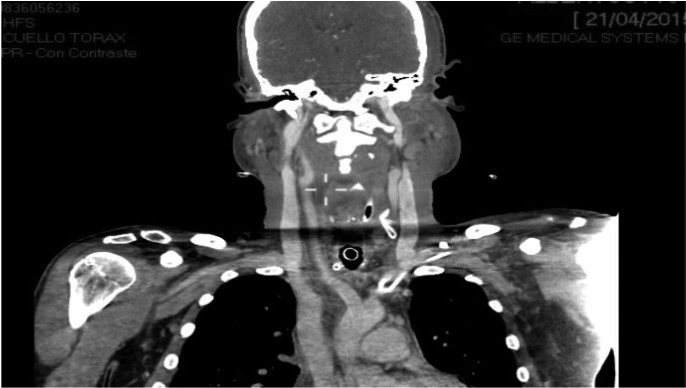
CT images that show the pus which spread from the tooth 48 to the mediastinum.
